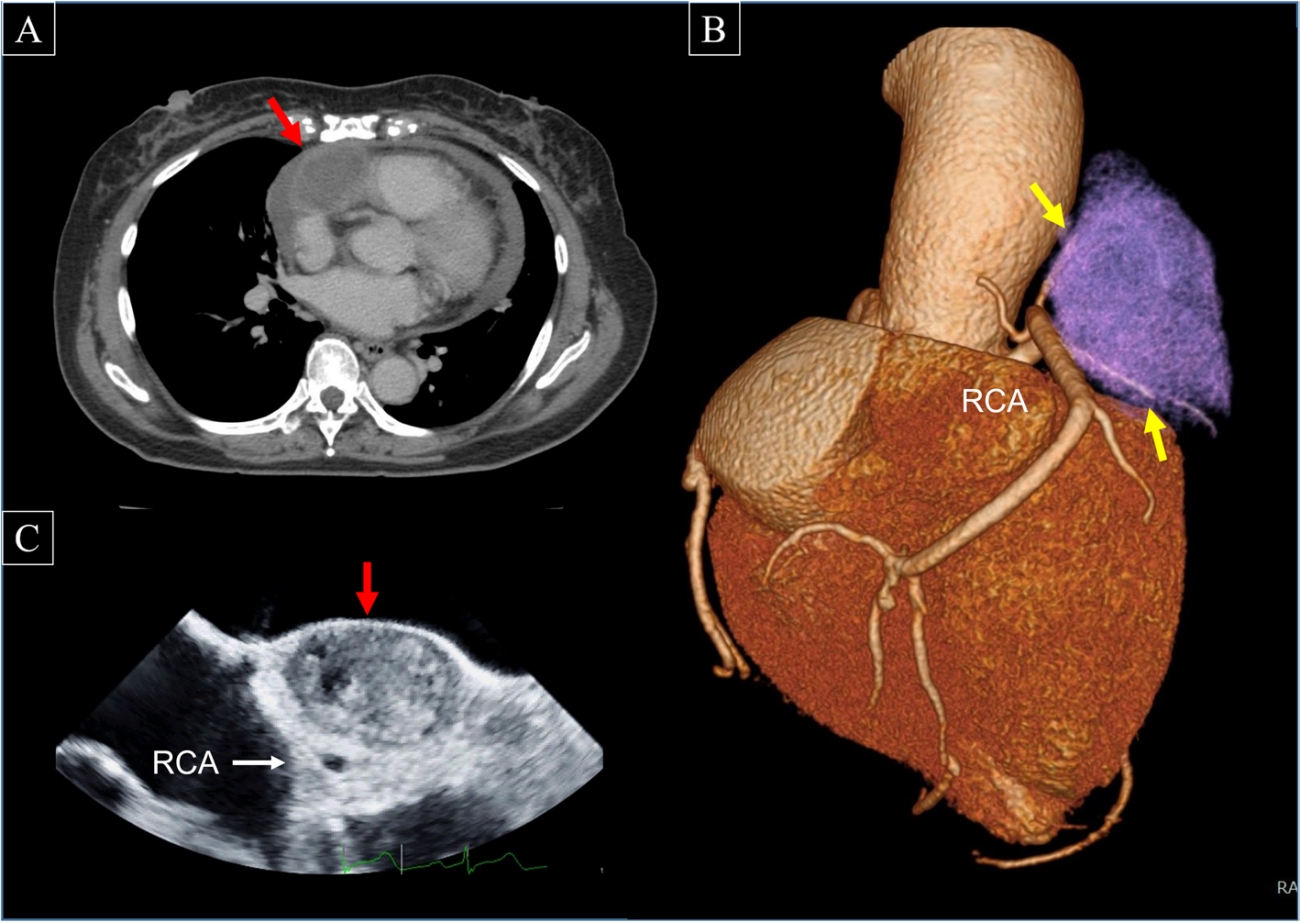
A Computed tomography revealing a 44-mm mass lesion (red arrow) occupying the front of the right atrium. B Multidetector computed tomography revealing two branches of the proximal right coronary artery surrounding the lesion (yellow arrows). C Epicardial direct echocardiography demonstrating an oval mass with high echoic matrix (red arrow)
